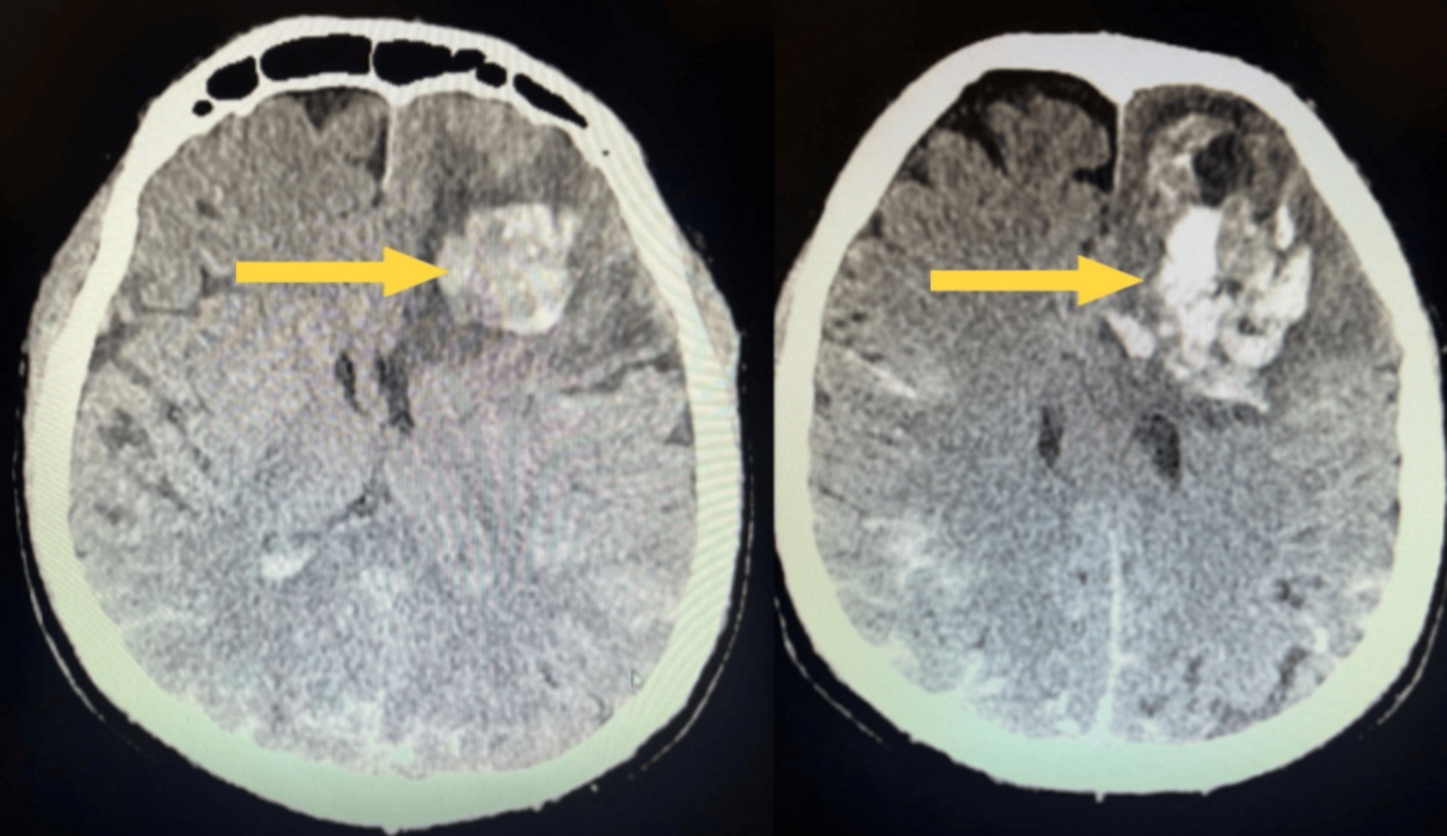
Follow-up CT scan of the head performed on the second day after injury Follow-up CT scan on day 2 showing increased white matter edema with greater mass effect and subarachnoid hemorrhage with abundant blood in the parietal and occipital sulci.
